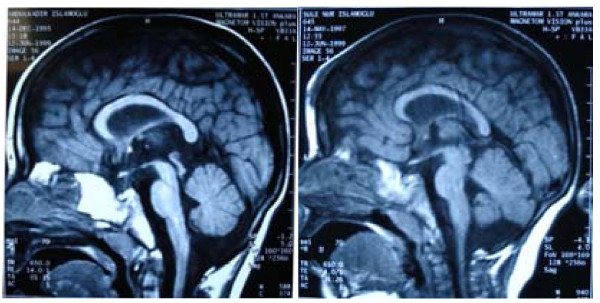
Cranial MRI showing diffuse cerebral atrophy with a relatively normal sized corpus callosum, relatively large lateral ventricules and normal sized brain stem and cerebellum.
